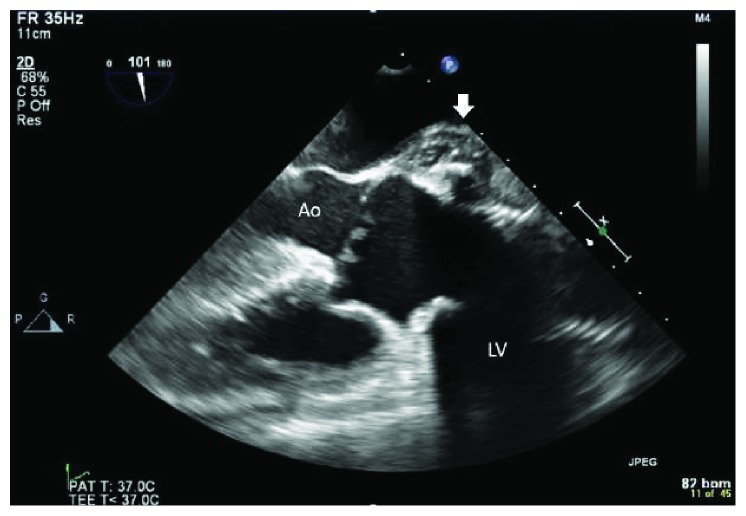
Mid-esophageal long-axis view of the heart with Doppler in the transesophageal echocardiogram showing severe aortic regurgitation.
